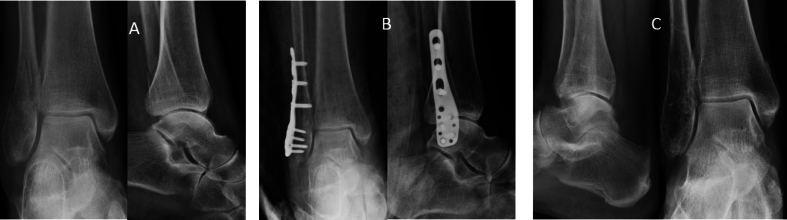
A 44B1 ankle fracture. A: pre-operative x-rays. B: after surgery x-rays. C: seven years follow-up x-rays.
